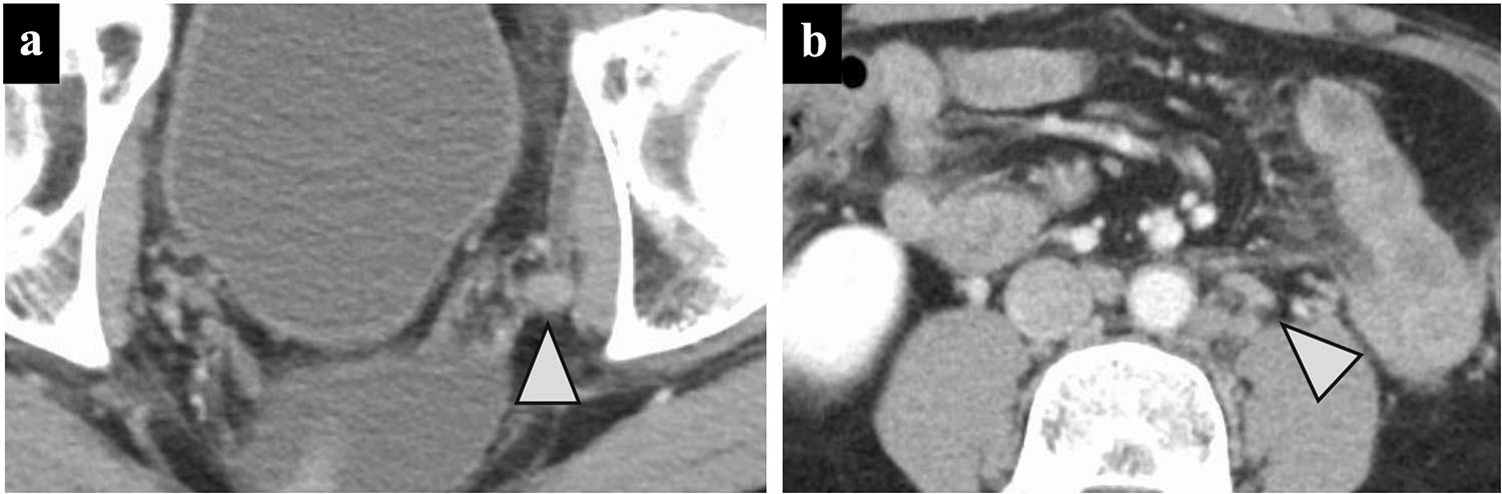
Contrast-enhanced CT findings at the time of early recurrence at the lymph nodes. a The metastatic left lateral lymph node was enlarged (arrowhead). b The para-aortic lymph nodes were enlarged (arrowhead)
